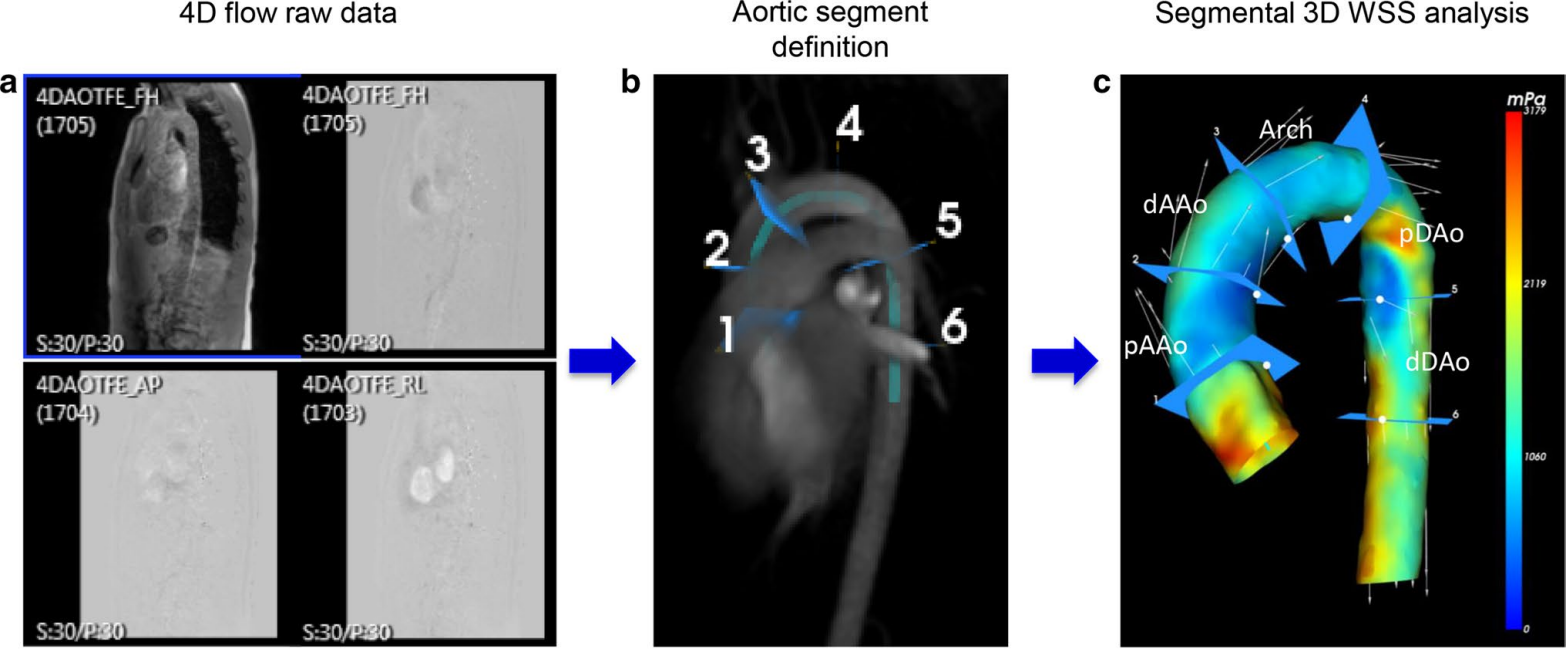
Aortic 4D flow MRI processing and analysis. a 4D flow MRI raw data including anatomical and flow data. b Automatic segmentation of 3D aortic volume after manually defining start and endpoint of the thoracic aorta and aortic segment definition. c 3D color-coded aortic segmentation representing WSSmax distribution for one systolic cardiac phase
